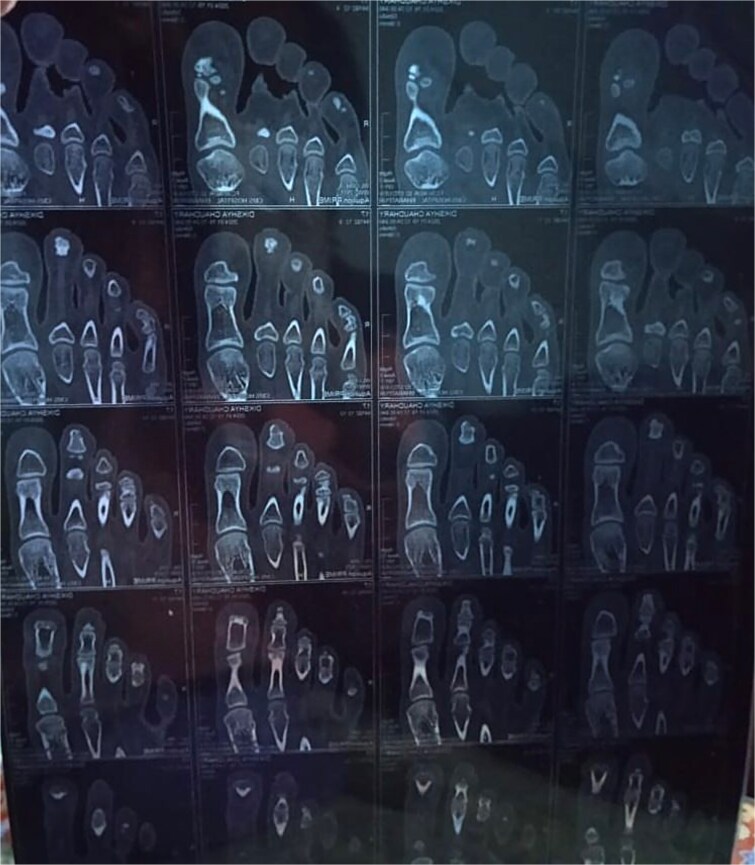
CT scan of right foot revealing a linear undisplaced fracture over distal phalanx of second toe.
